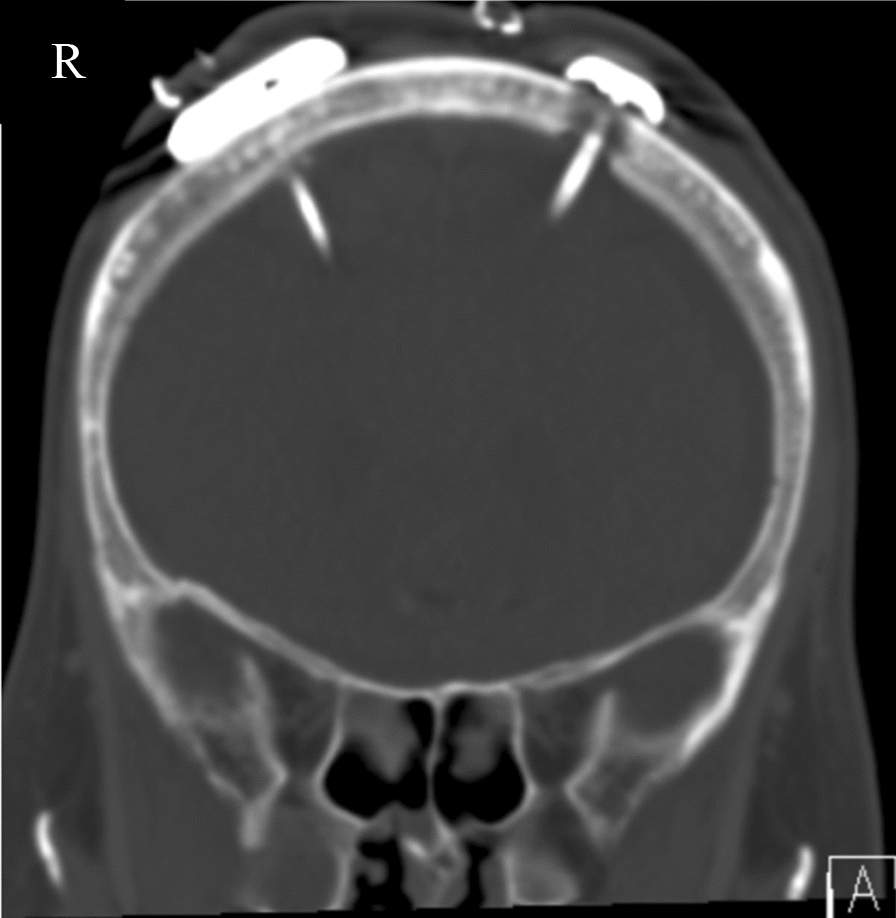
Coronar reformation of a cerebral CT after implantation of a NEUROVENT^®^-P-tel probe through a right frontal burr-hole and a sensor reservoir^®^ connected to a ventricular catheter through a left frontal burr-hole. (Institute for Radiology and Neuroradiology at the Unfallkrankenhaus Berlin, Director Prof. Dr. med. S. Mutze)
